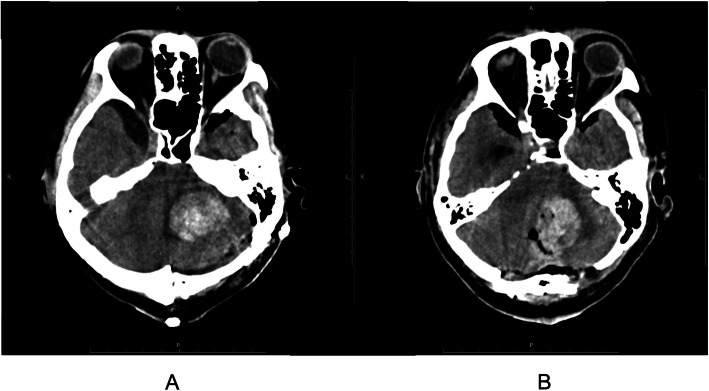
Postoperative intracranial hematoma. Postoperative brain computed tomography showing intracranial hematoma. Hematoma in the left cerebellopontine angle in case 1 (a) and case 2 (b) was detected by postoperative bran computed tomography examined after the onset of bradycardia in the ICU
